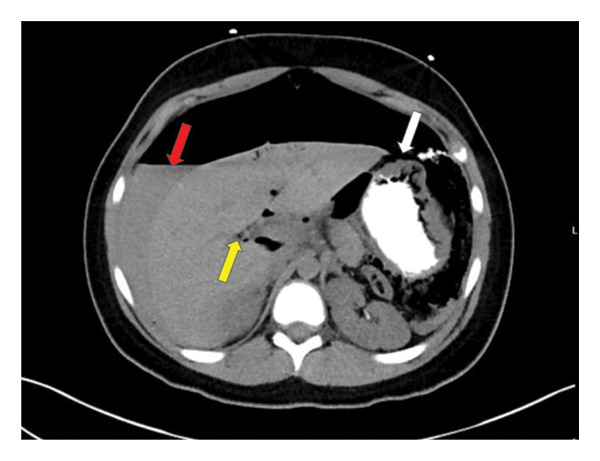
Abdominal computed tomography (CT) scan with PO contrast, axial view, demonstrates discontinuity of the stomach at the level of the fundus along the greater curvature, indicating rupture (white arrow). Large pneumoperitoneum is seen, and a large amount of free fluid containing diluted ingested oral contrast (red arrow) is observed. There is a small amount of peripheral air within the left hepatic lobe, indicating portal venous gas (yellow arrow).
